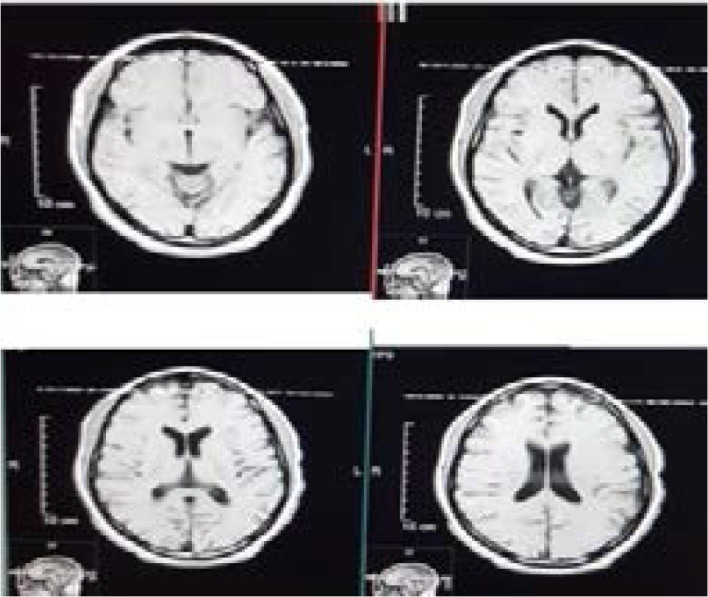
Frequency artifact as a result eye motion during acquisition of brain MRI.
